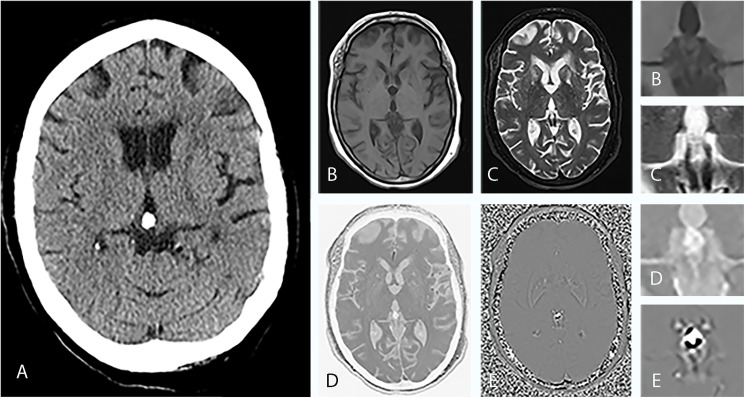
Imaging findings of a 55-year-old woman with a calcified pineal gland. (A), CT shows a sharply defined oval-shaped pineal calcification with a diameter of 11 mm. In axial T1-weighted MRI (B) and in axial T2-weighted MRI (C) it is hardly possible to demarcate the calcified area against the surrounding tissue. In conventional MRI, it is not possible to reliably identify the hypointense foci as calcifications. The inverted SWMR magnitude image (D) and the phase image (E) show well-defined focal hyperintensities in the pineal region area. While the image information solely derived from the magnitude image is not superior to conventional MRI sequences, the combination of SWMR magnitude and phase image allows for a clear and reliable identification of diamagnetic calcifications. Magnified images are provided for (B), (C), (D) and (E). As CT and MRI of the brain have diverged reference lines with the bicommissural line used as a convenience standard for MRI and the orbitomeatal line used for CT, the slice angles vary accordingly.
